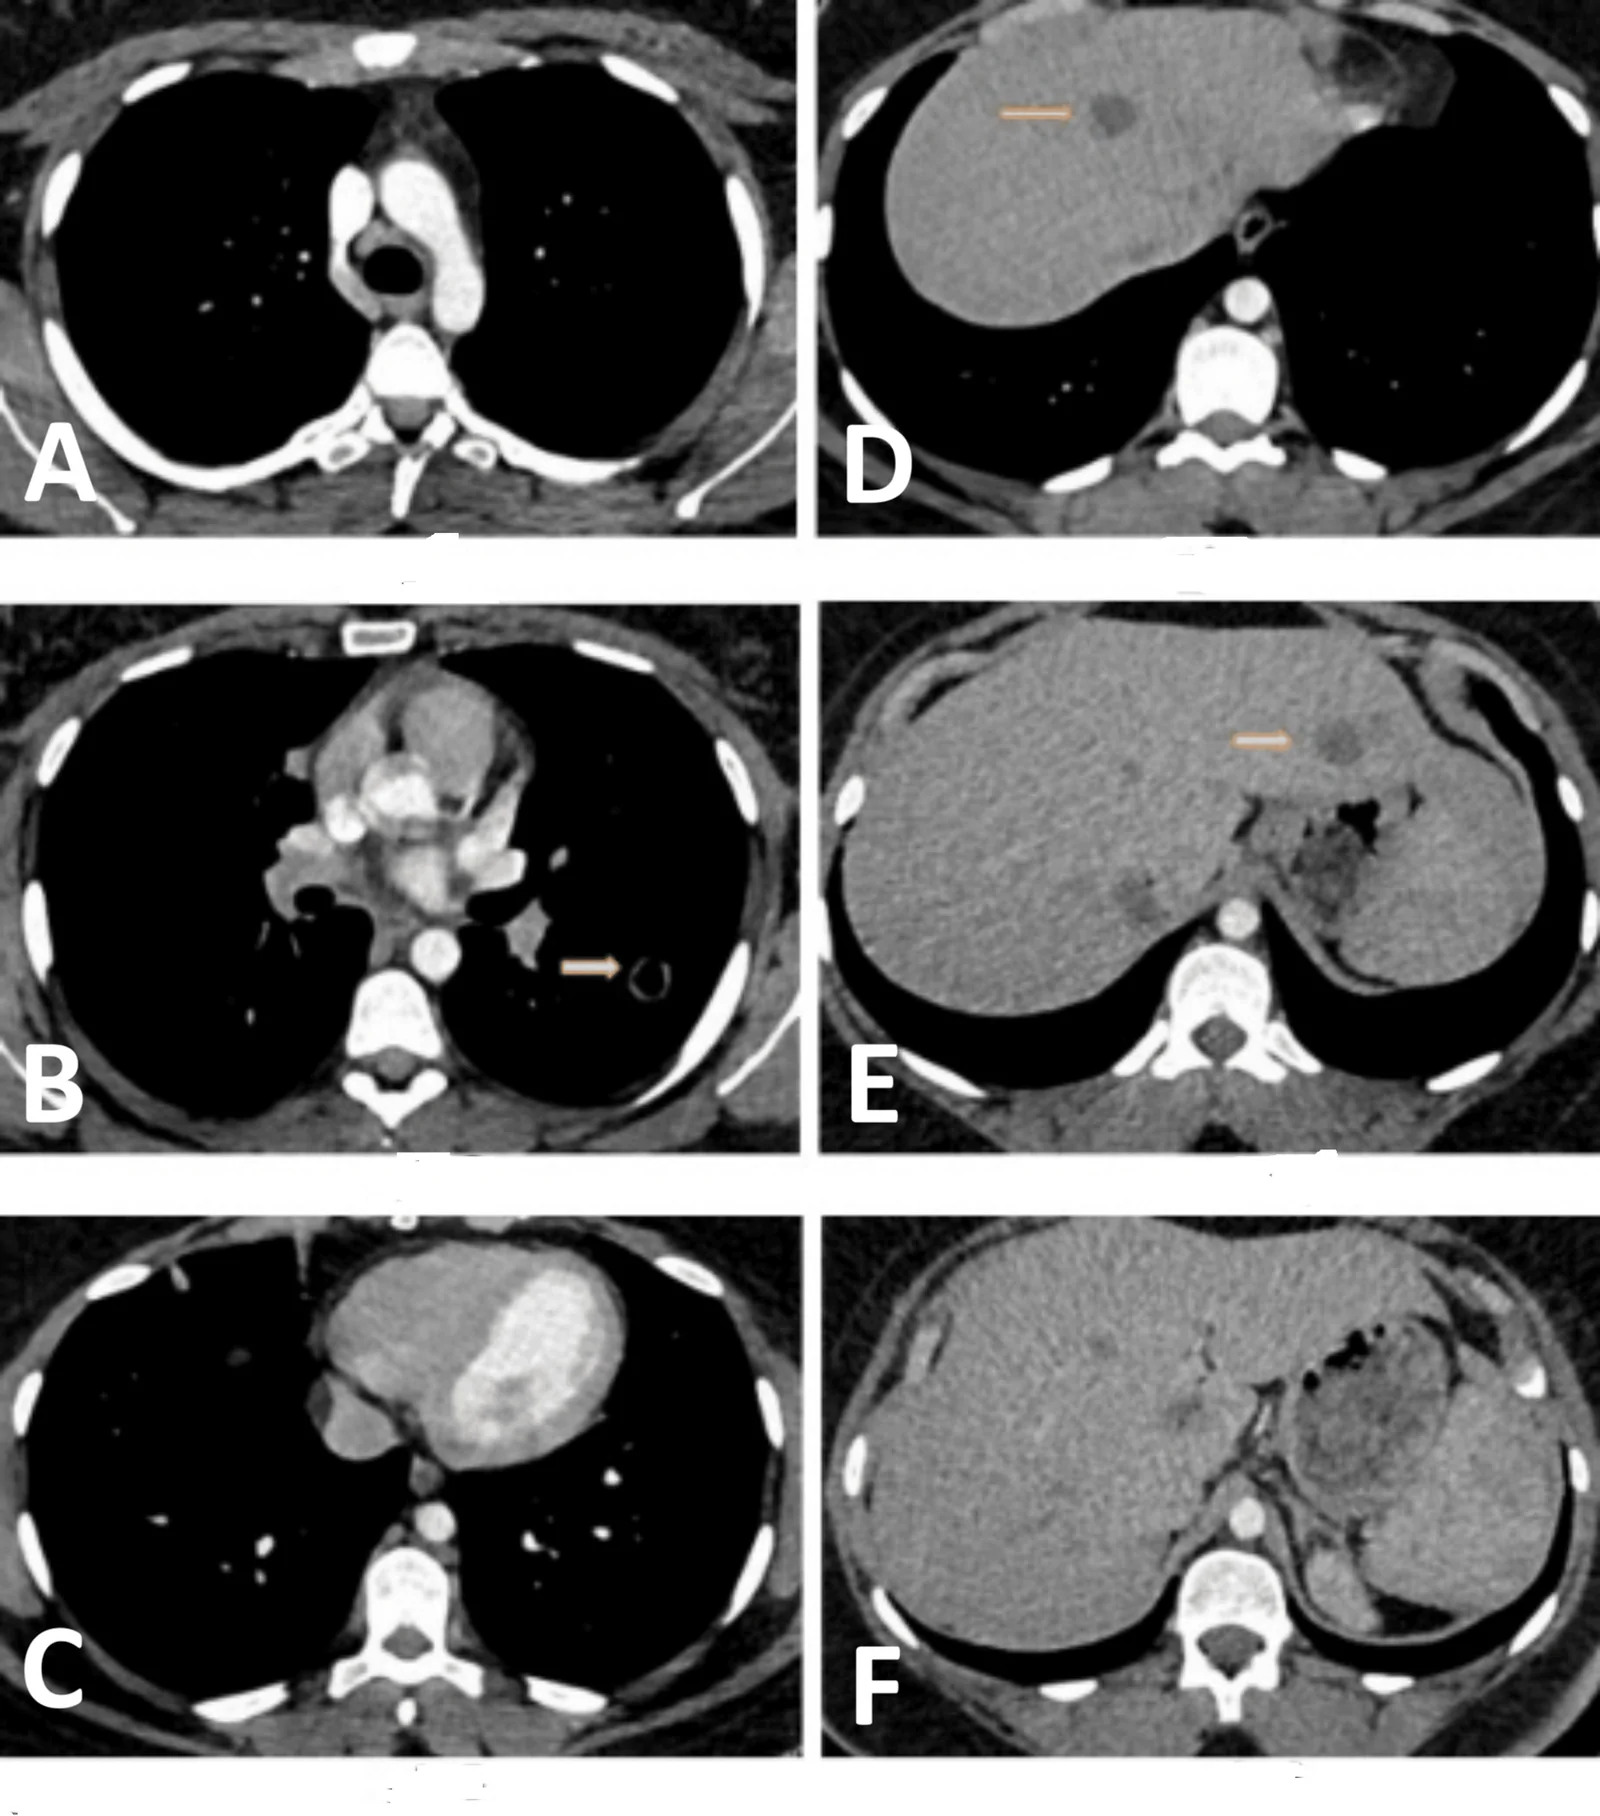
CT scan of the chest and abdomen after five weeks of treatment: (A-C) cross-sectional images of the upper and mid-lung fields; (D-F) cross-sectional images of the upper right and left liver lobes A repeat scan showed resolution of the pulmonary cavitary and liquefactive changes, with stable hepatic *Echinococcus *cysts. (B) The arrow indicates cavitary transformation consistent with treatment-related cyst degeneration. (D, E) The arrows indicate multiple hepatic hypodense lesions that remained stable.
